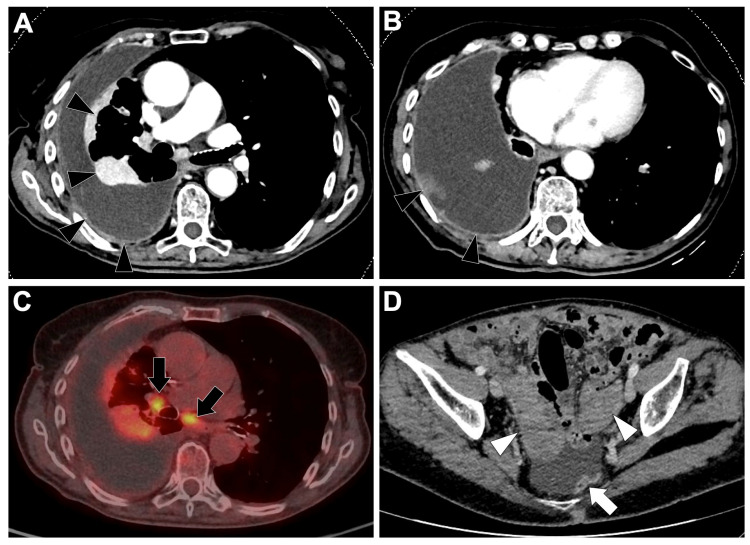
Representative baseline CT imaging findings in patients with NTRK-rearranged lung adenocarcinoma (LUAD). A 76-year-old never-smoking woman presented with a large right pleural effusion and pleural metastatic deposits (black arrowheads) (A,B). 18F-FDG PET/CT demonstrated metabolically active mediastinal lymphadenopathies (black arrows) (C). Pelvic CT revealed bilateral ovarian metastases (white arrowheads), peritoneal effusion, and peritoneal tumor deposits (white arrow) (D).
